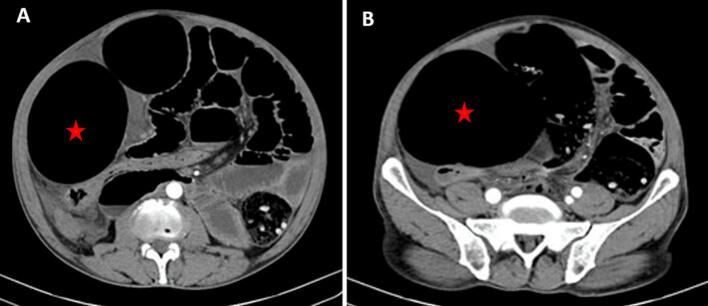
A - The abdominal CT scan revealed a whirl sign and significant distention of the haustral sigmoid loop, measuring 12 cm in diameter with parietal thinning. B - Sigmoid colonic loop with air-fluid level and beak appearance of the efferent limb of volvulus and dilated small bowel with poor contrast effect (Red star: dilated loop)
